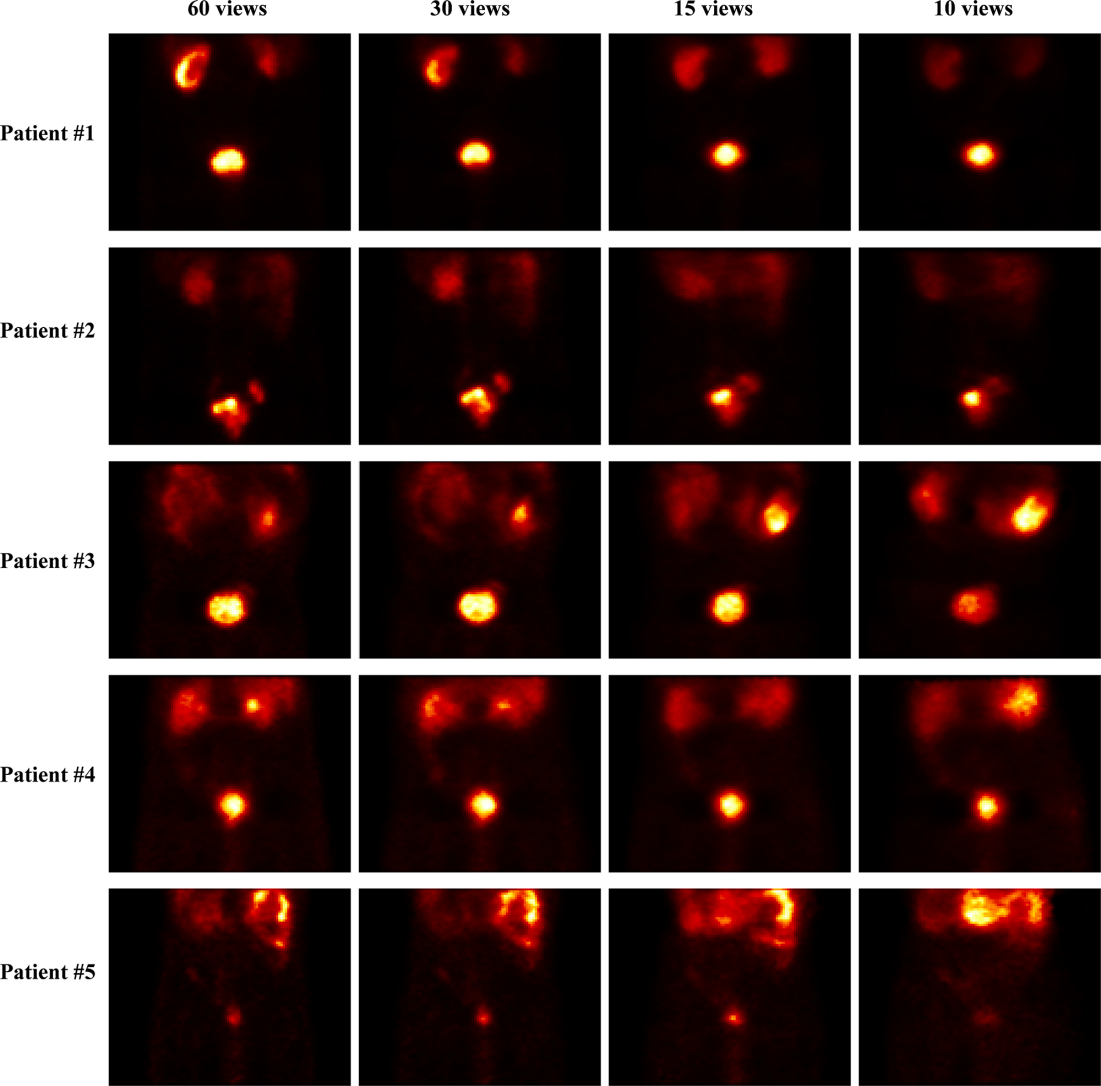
SPECT images with the OSEM algorithm at 60, 30, 15, and 10 views (patients number 1 to number 5). OSEM, ordered subset expectation maximization; SPECT, single photon emission computed tomography.
